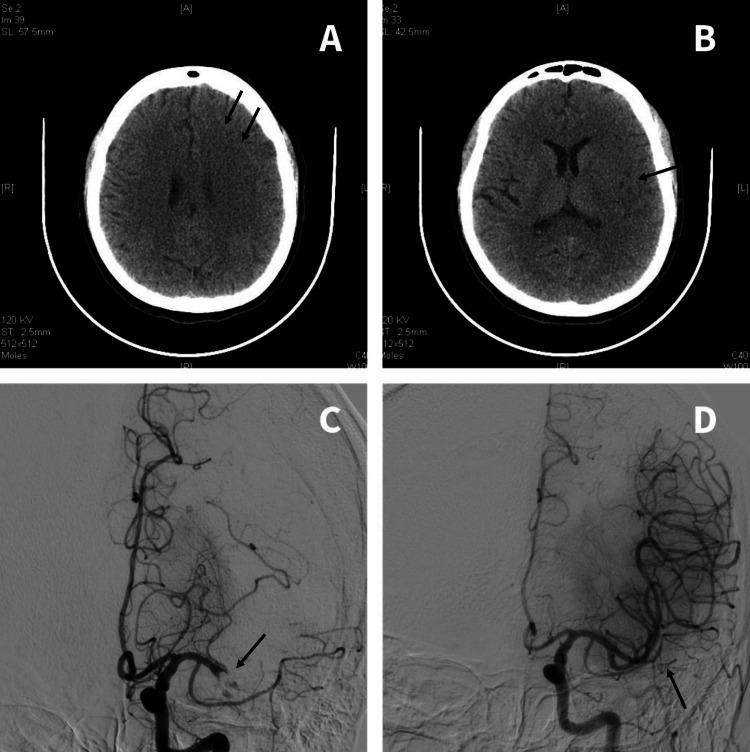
Intracranial imaging. (A) Plain head computed tomography scan revealing subtle decreased attenuation of the grey-white matter junction in the left frontal lobe (black arrows). (B) Plain head computed tomography scan revealing subtle decreased attenuation of the grey-white matter junction in the left insular regions (black arrow). (C) Computed tomography angiography revealing occlusion of the left middle cerebral artery at proximal M1 segment (black arrow). (D) Final angiographic result showing successful reperfusion (black arrow) and a final thrombolysis in cerebral infarction (TICI) score of 2b.
